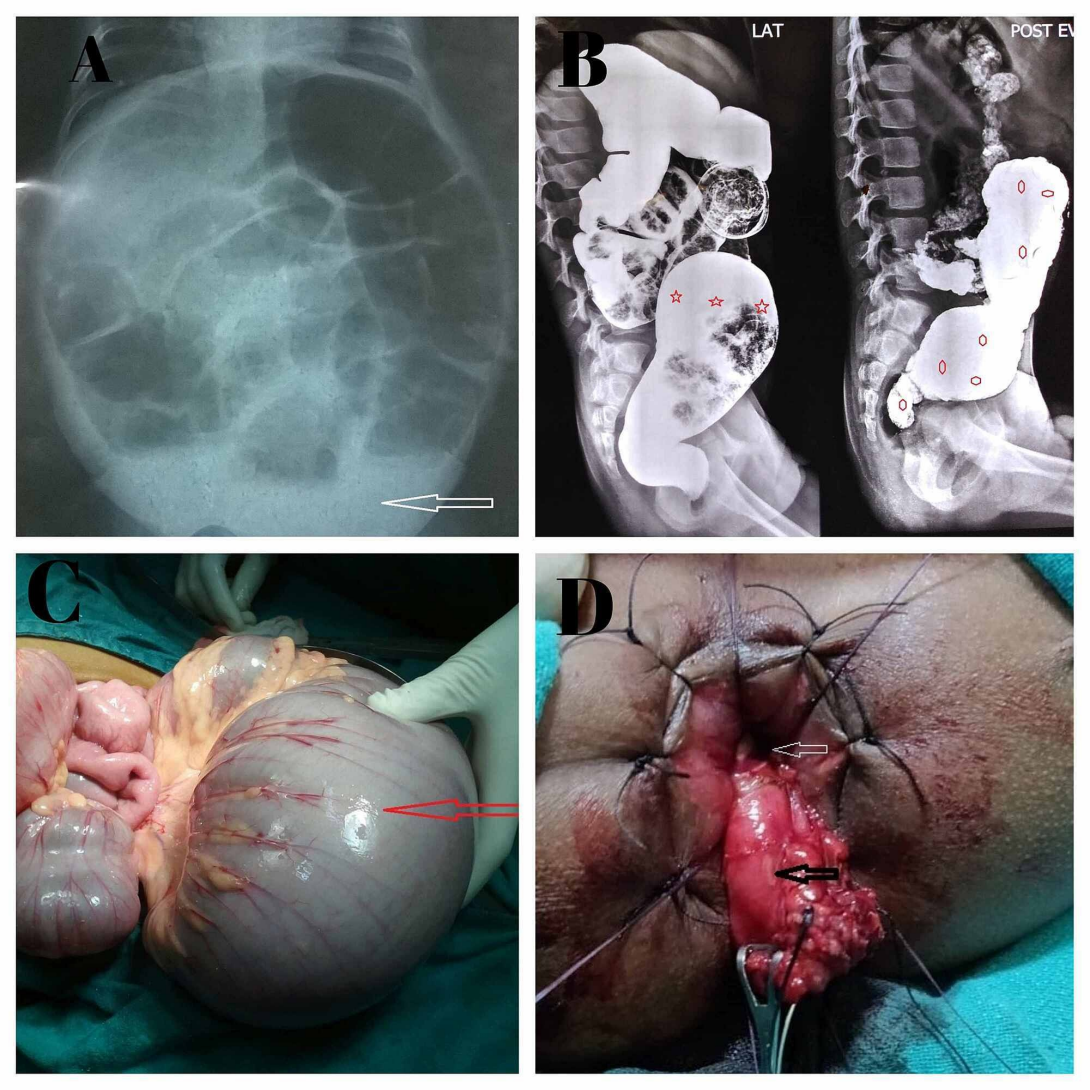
Image showing radiological and surgical features of HD. (A) Plain X-ray: paucity of air in the pelvic cavity (white arrow); (B) Contrast enema: sigmoid colon (star shape) is more dilated than rectum (reversal of rectosigmoid index) and 24-hour delayed film holding of dye in bowel (hexagonal shape); (C) intra-operative dilated sigmoid colon; (D) intra-operative retro rectal (white arrow retro rectal space) pull through (black arrow pull through bowel). HD: Hirschsprung’s disease.
